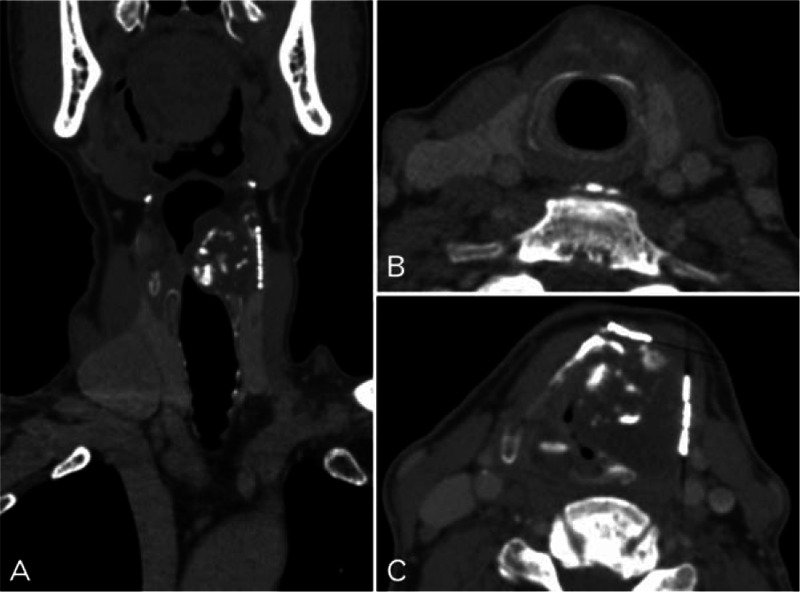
Contrast-enhanced computed tomography images of the laryngeal chondrosarcoma. Coronal (A) and axial (B and C) contrast-enhanced computed tomography images at bone windows show a mass from the thyroid cartilage, with a calcified matrix. B and C show that the cricoid cartilage and right arytenoid cartilage are free of damage.
